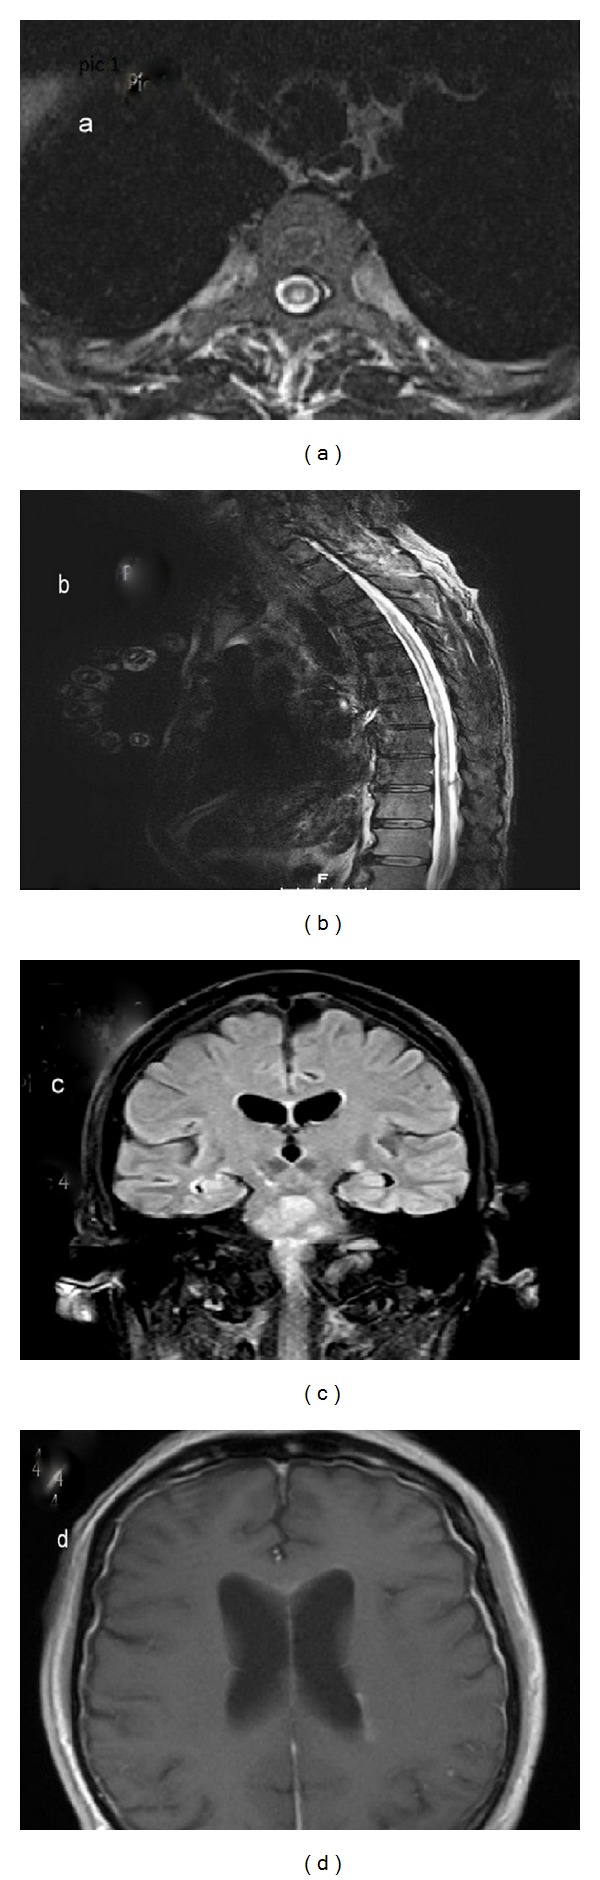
(a) Axial T2W of thoracic spinal cord and (b) sagittal T2W of thoracic spinal cord demonstrating intramedullary central T2 hyperintensity which extends from T2 to T10 level. (c) Coronal FLAIR demonstrating hyperintensity along the walls of the lateral and third ventricles, brainstem, and lateral geniculate bodies; on T1 postcontrast imaging (d) mild enhancement is noticed along the walls of the lateral ventricles.
